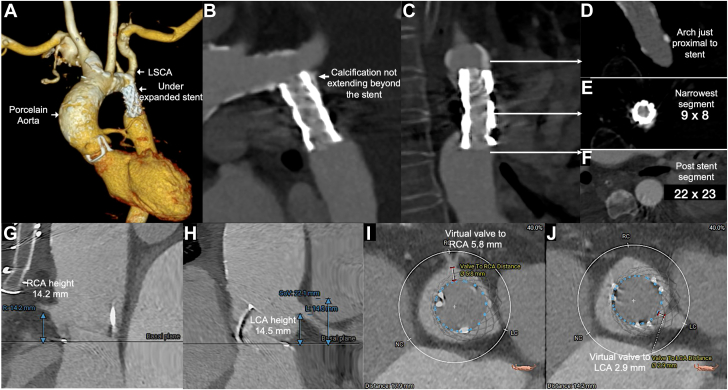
Cardiactomography aortogram. (A) A 3-dimensional reconstructed model of aorta showing diffusely calcified porcelain ascending aorta (arrow) with extension to arch. The coarctation stent is not fully expanded (arrow) extending from the isthmus to nonstenotic segment of proximal descending thoracic aorta. (B) Speckle of calcification (arrow) is noted posterior to the stent just beyond the left subclavian artery (LSCA) and is fully covered by the stent. (C, D) The calcification cranial to stent is localized to lateral walls of the distal transverse arch and are unlikely to interact with balloon dilation. The narrowest internal diameter of the stent was 8.0 × 9.0 mm (E) and the aorta distal to stent was 22.0 × 23.0 mm (F). Computed tomography assessment of aortic valve: computed tomography scan showing right coronary artery (RCA) height of 14.2 mm (G), left coronary artery (LCA) height of 14.5 mm, sinus of valsalva (SOV) height of 22.1 mm (H), virtual valve to right coronary artery of 5.8 mm (I), and virtual valve to left coronary artery of 2.9 mm (J). L, left coronary artery, LC, left coronary cusp, NC, non coronary cusp, R, right coronary artery, RC, right coronary cusp.
